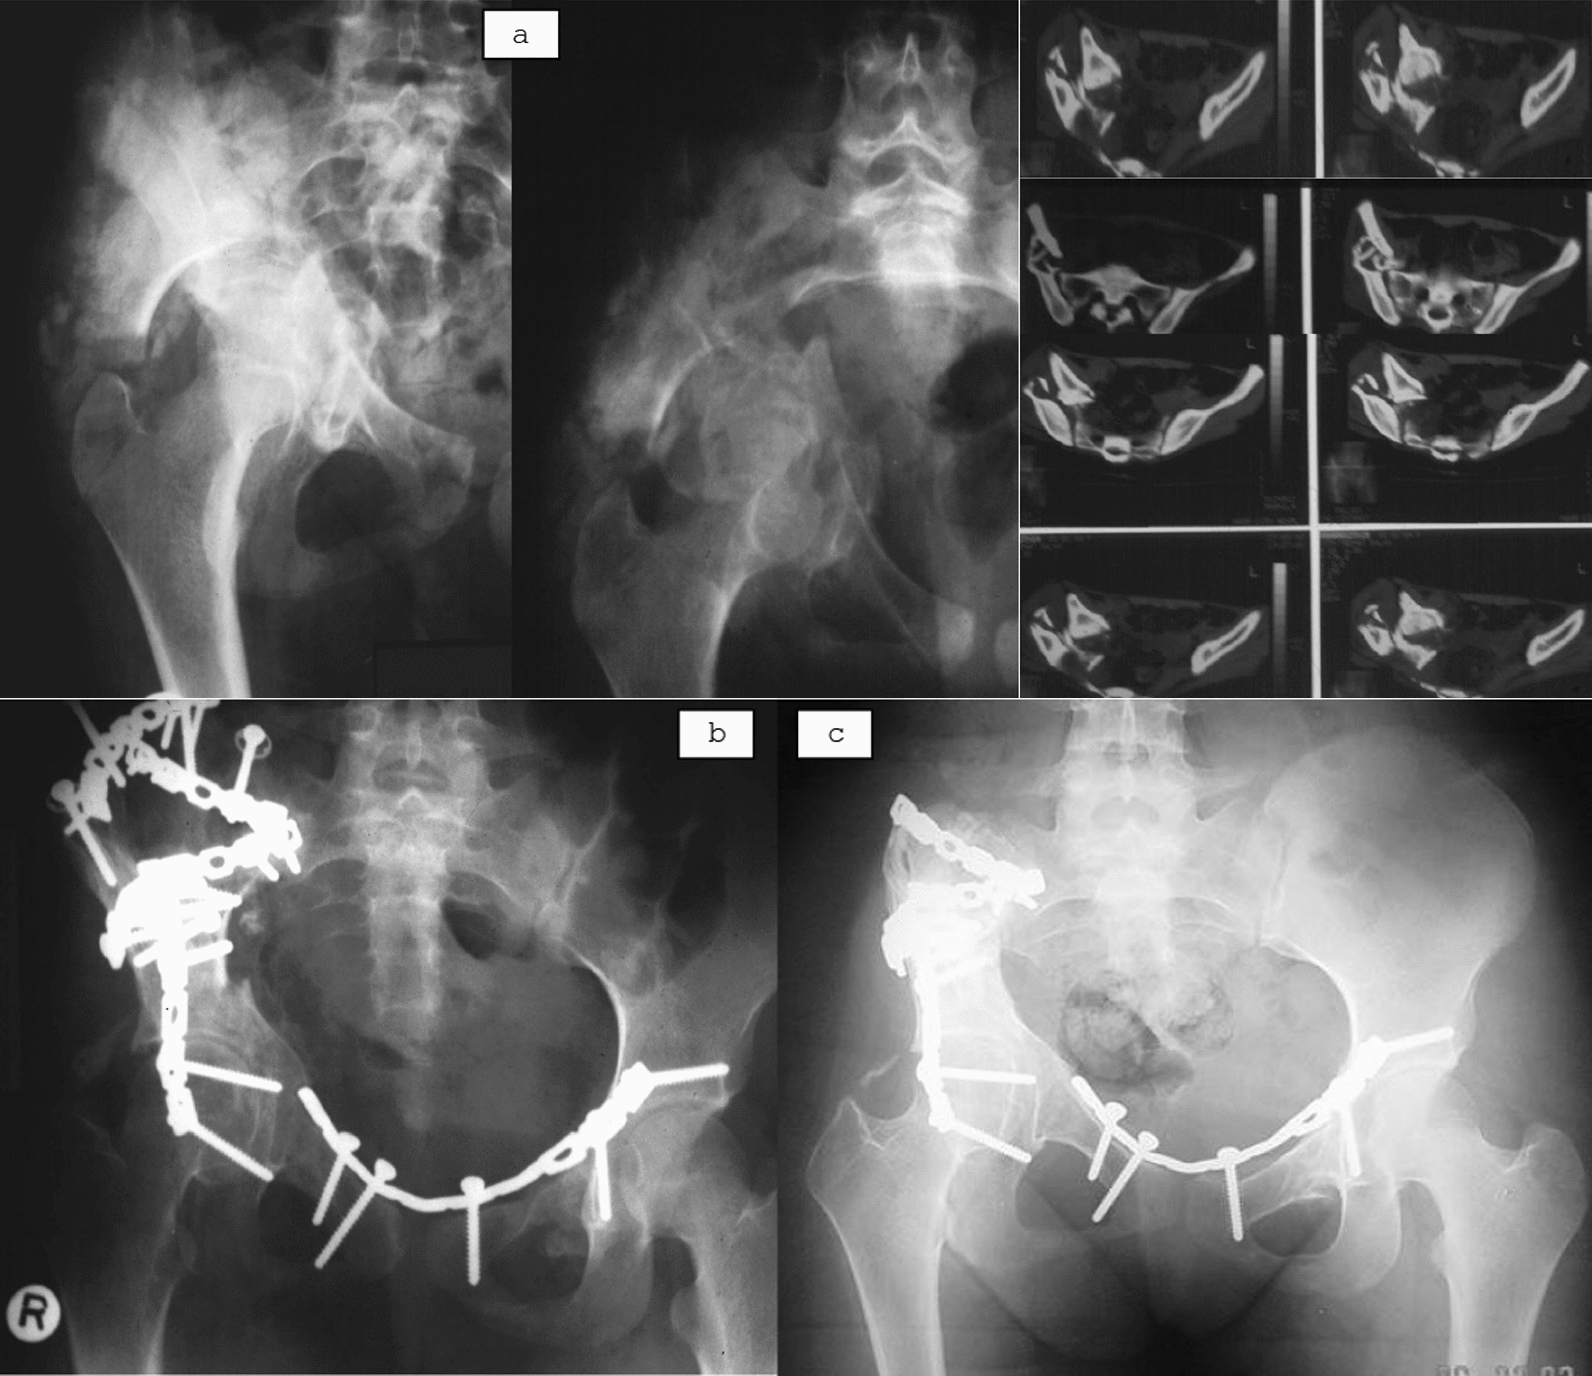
A 14-year-old girl with an 8-week-old right neglected associated both-column fracture. a Preoperative radiograph and Axial CT image showing severely displaced fracture with central femoral head subluxation and early callus formation. b 1 year follow up radiograph showing anatomical reduction and stable fixation with a congruent hip joint. c Final 6-year follow-up AP radiograph demonstrating excellent joint remodeling, maintained reduction, and implant removal around the iliac crest
